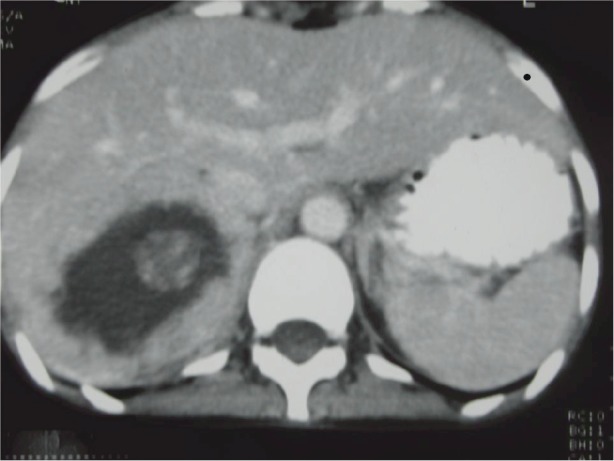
CT abdomen. Huge right suprarenal mass measuring 16.5 × 6.5 × 8.7 cm.
